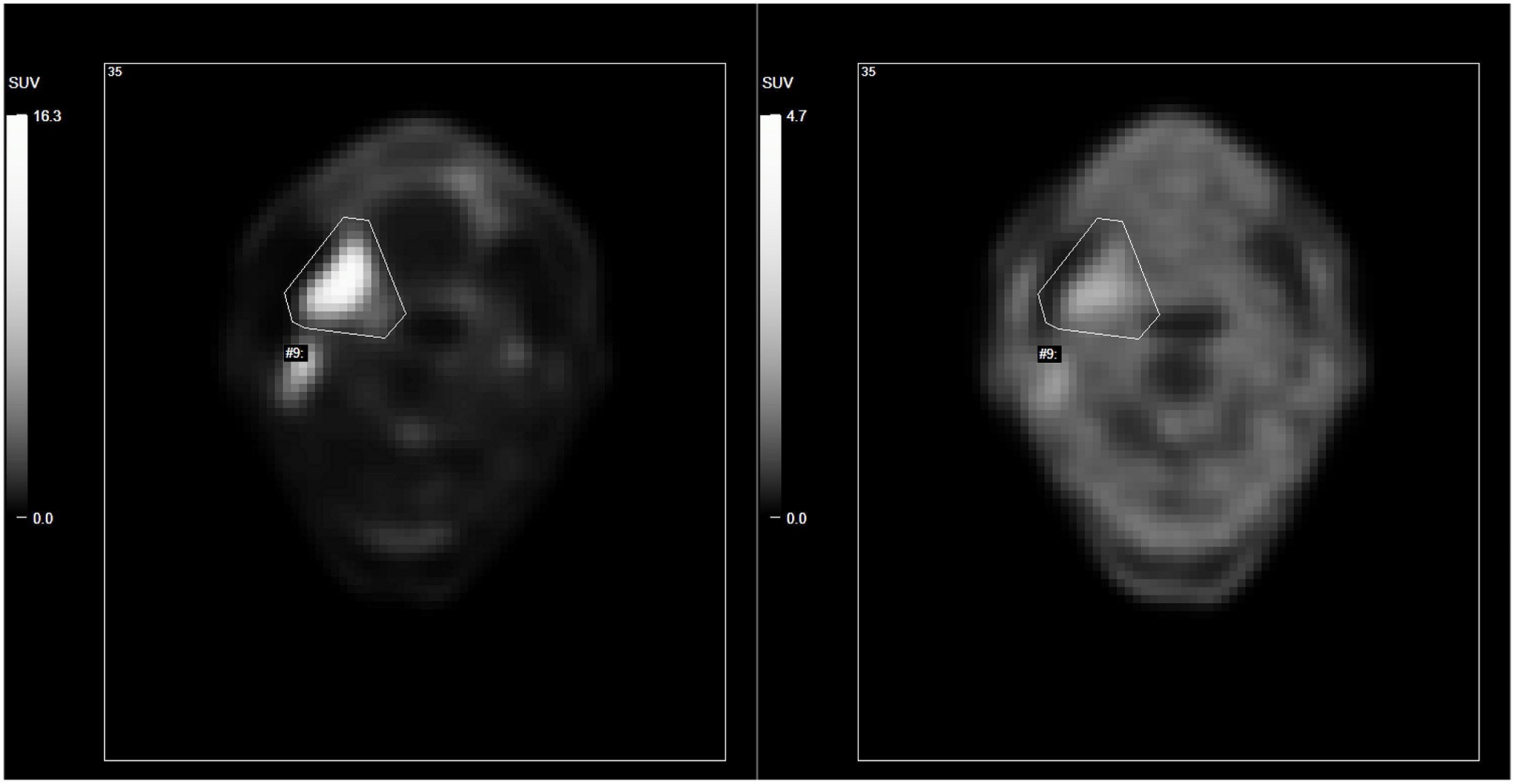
Side by side comparison of FDG (left) and FMISO (right) PET image of a representative patient with a SCC on the right side surrounded by the manual ROI. Note: different contrast ratio selected for each PET image.
